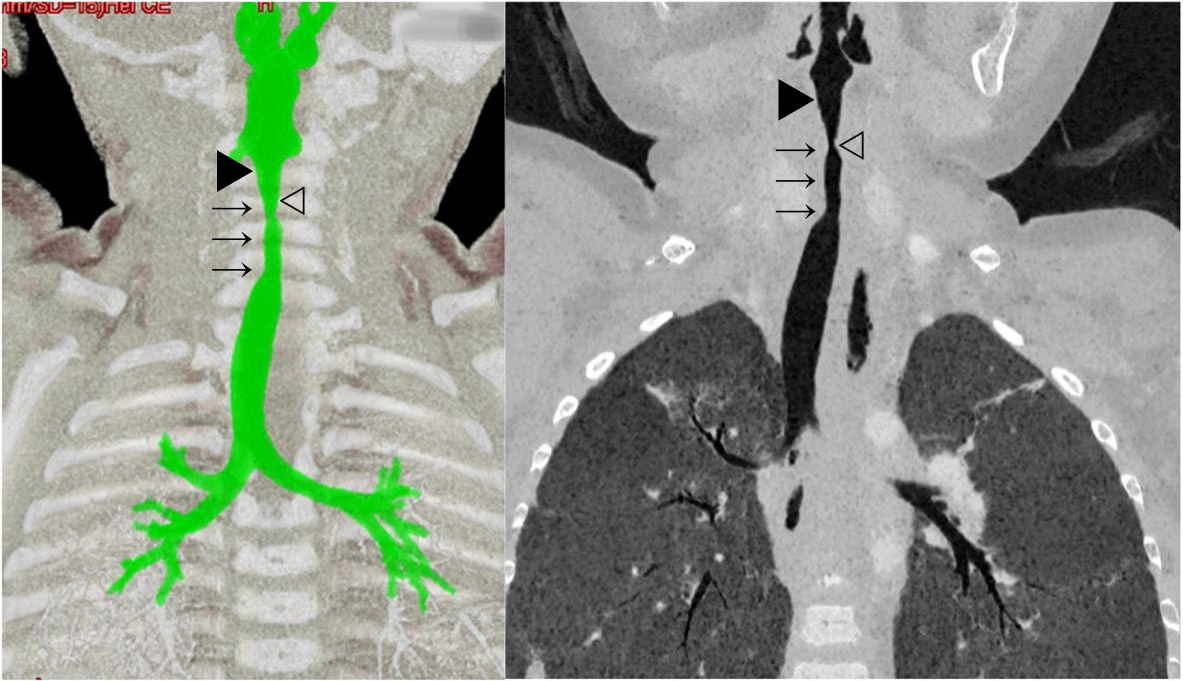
CT images. The length of stenosis (arrow) was 1.2 cm. Stenosis started at approximately 7.5 mm below the vocal cords (black triangle). At the narrowest part (white triangle), the anterior-posterior diameter was approximately 4.0 mm and the transverse diameter was 1.7 mm. Below the stenosis, the diameter of the trachea was 7 mm, which is normal
